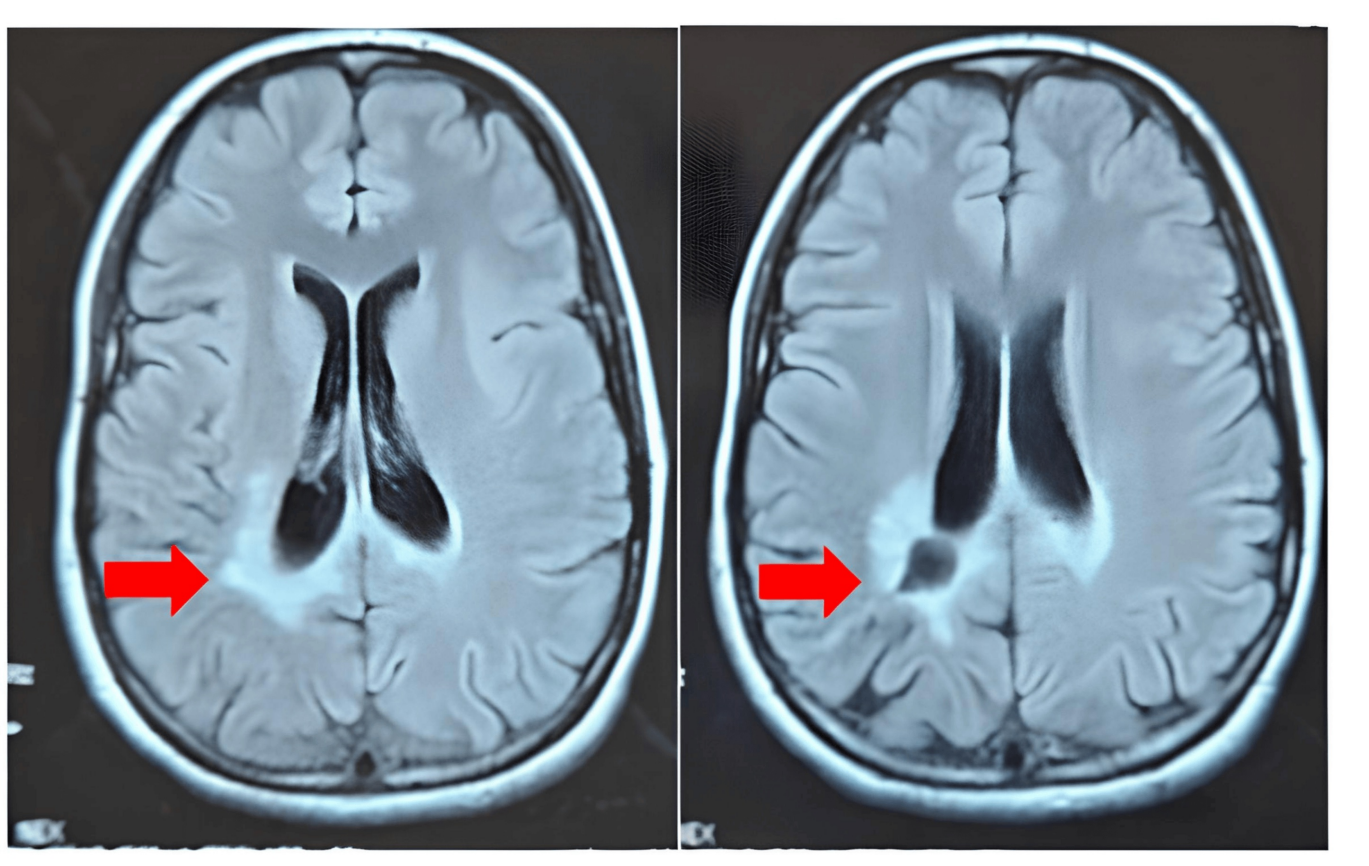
Axial brain MRI FLAIR sequences obtained at 18 months follow-up. Axial brain MRI FLAIR sequences obtained at 18 months follow-up showing near-complete regression of the previously identified lesion in the left parietal region (red arrows). The hyperintense signal seen in earlier scans has markedly decreased, with only minimal residual gliotic changes remaining. FLAIR: fluid-attenuated inversion recovery.
